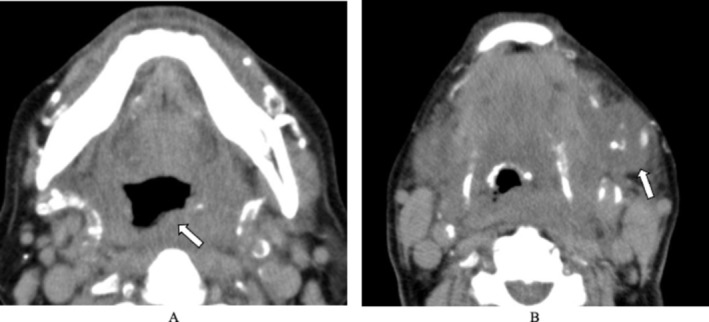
Contrast‐enhanced CT images on the first hospital day. CT images showing airway obstruction due to swelling of the left pharynx (A, arrow) and the left submandibular gland (B, arrow).
